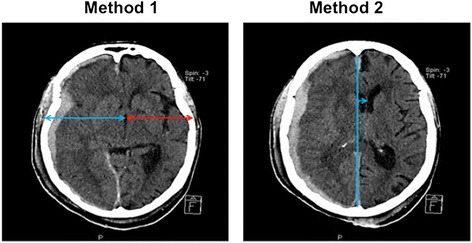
Example of MLS measure with CT scan: on the left, method 1 (in the same plane as sonography through the third ventricle), and on the right, method 2 (measuring the distance between the ideal median line and the septum pellucidum). CT, computed tomography; MLS, midline shift.
